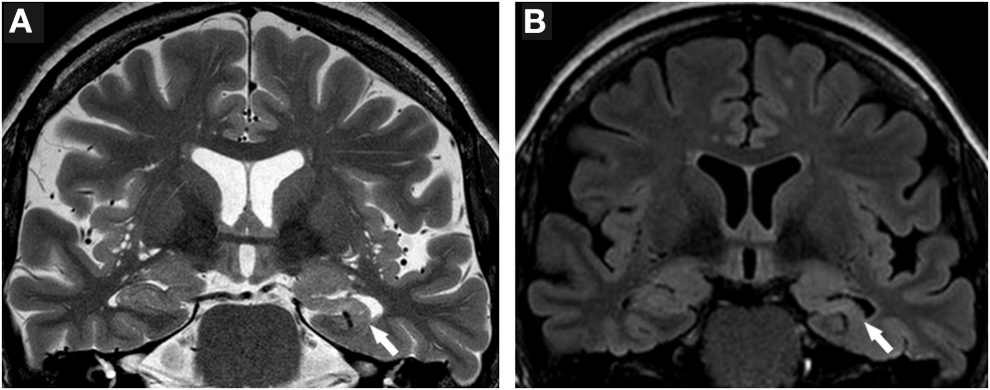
Neuroimaging findings in Patient #4. (A) T2-weighted brain MRI scans reveal hippocampal volume loss and a markedly disturbed inner structure of the hippocampus on the left side (white arrow). (B) FLAIR images further highlight volume loss and signal hyperintensity of the left hippocampus (white arrow). Structures of the right medial temporal lobe do not show pathologic signal alterations.
